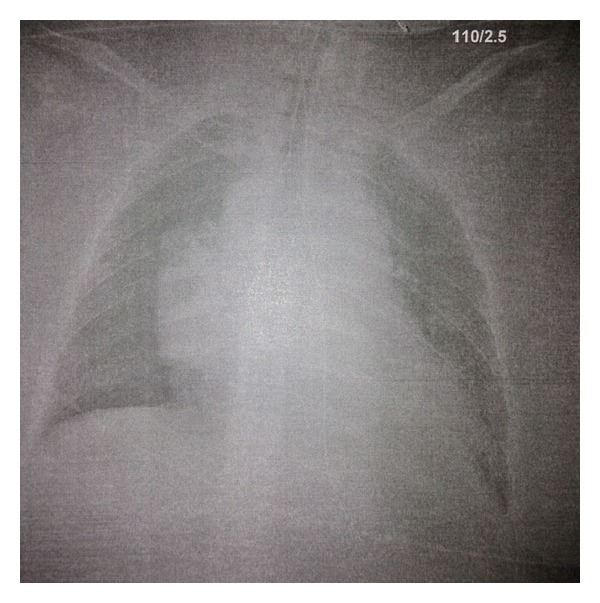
Chest X-ray showing large mediastinal mass.
